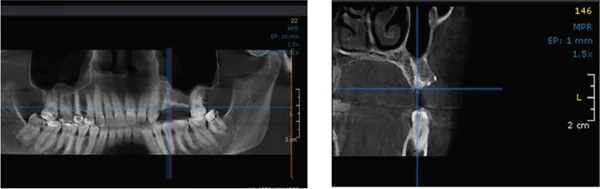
Radiographic examination after extraction and socket preservation, demonstrating mature regenerated bone at the 6‐month healing period.
